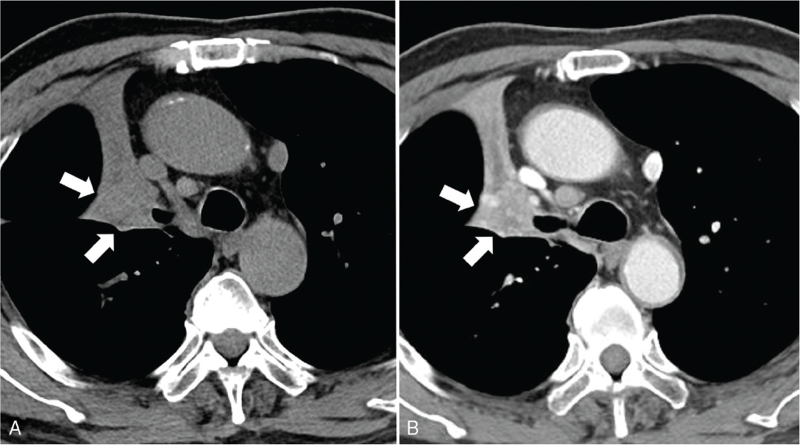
A 74-year-old man with basaloid squamous cell carcinoma of the lung. Unenhanced (A, B) and enhanced (B) CT scan shows enhancing mass obstructing right upper lobar bronchus with (arrows) with distal atelectasis. CT = computed tomography.
